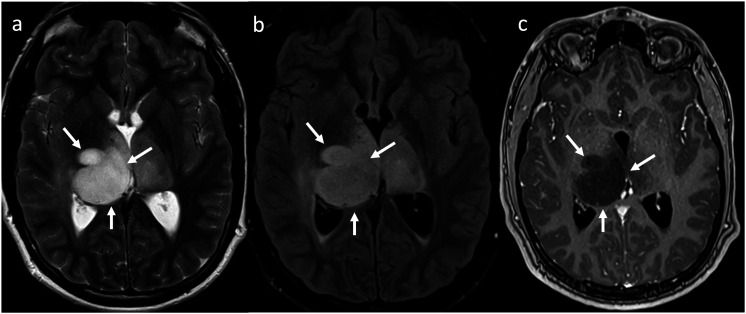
H3K27-altered diffuse midline glioma with partial T2FMM in a 19-year-old male. The portion of the mass that involves the right thalamus and basal ganglia (arrows) demonstrates mismatch, evidenced by homogenously bright signal on the T2WI (a), relatively hypointense signal on FLAIR except for a thin hyperintense peripheral rim (b), and lack of enhancement on postcontrast T1WI (c). The remainder of the lesion lacks mismatch, as it has lower signal on T2WI and lacks suppression on FLAIR sequence.
